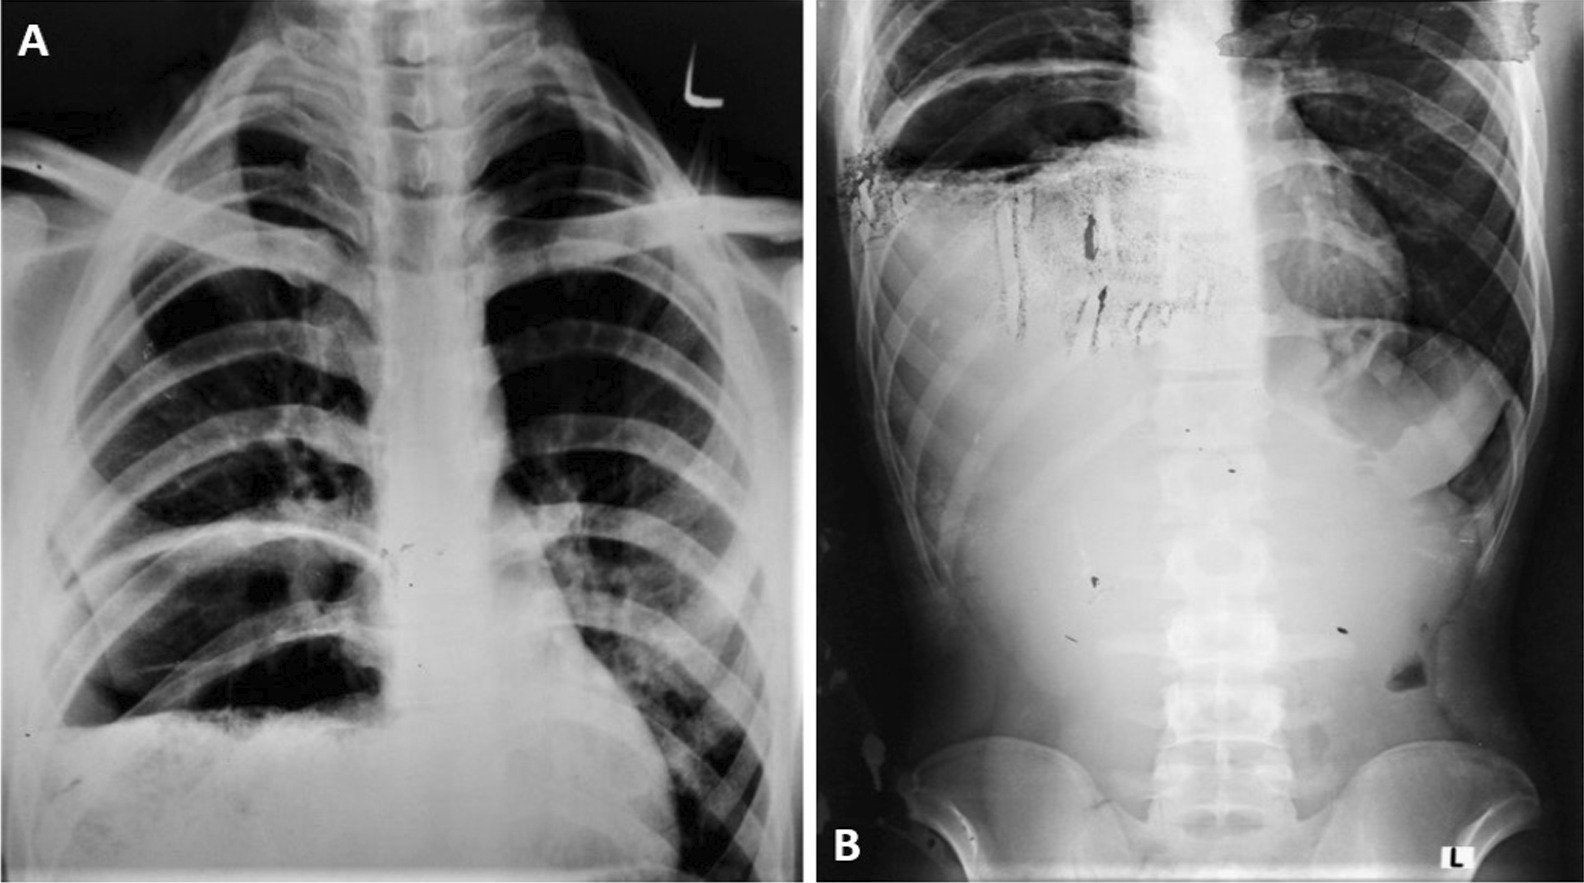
Chest X-ray revealed no gastric air shadow (A), but a large air fluid level in the right lower hemithorax with loss of normal diaphragmatic outline and paucity of bowel gases in the rest of the abdomen (B)
